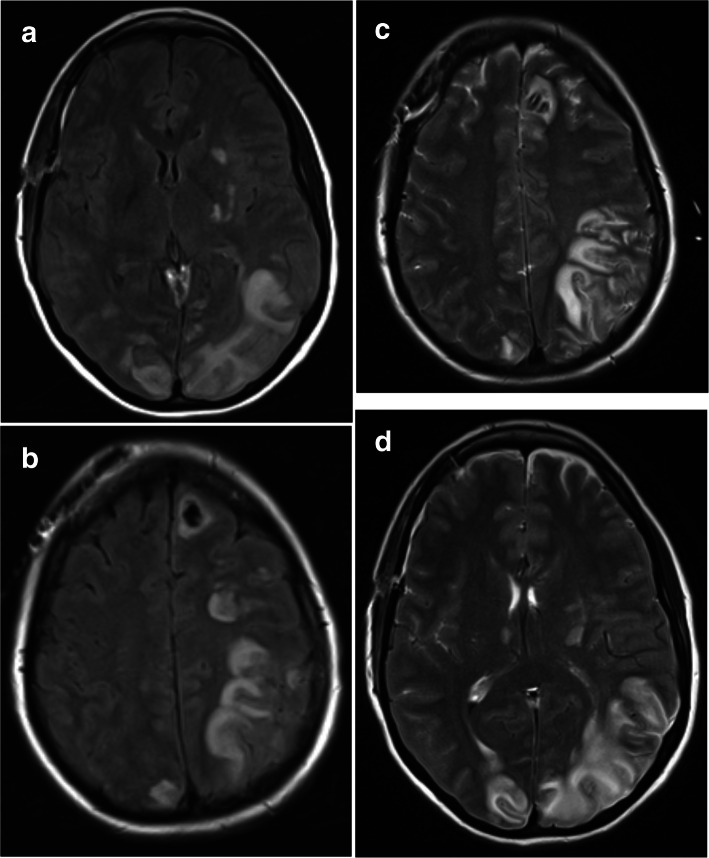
a Axial FLAIR demonstrating significant amount of predominantly left-sided parieto-occipital vasogenic edema. b Axial FLAIR demonstrating significant amount of predominantly left-sided parieto-occipital vasogenic edema. c T2 axial MRI demonstrating significant amount of predominantly left-sided parieto-occipital vasogenic edema. d T2 axial MRI demonstrating significant amount of predominantly left-sided parieto-occipital vasogenic edema
